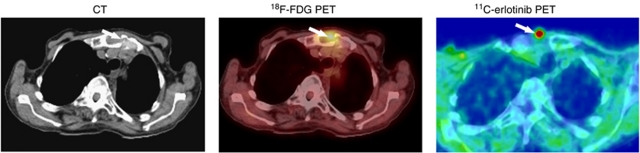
Accumulation of ^11^C-erlotinib in a bone metastasis from a NSCLC. Left: transaxial slices of contrast-enhanced CT; middle: ^18^F-FDG PET/low-dose CT; right: ^11^C-erlotinib PET/low-dose CT. A 79-year-old patient (no. 12) had NSCLC with metastasis to the sternum as shown on CT (arrow left figure). Both ^18^F-FDG and ^11^C-erlotinib accumulated in the metastatic lesion (arrows middle and right figures).
